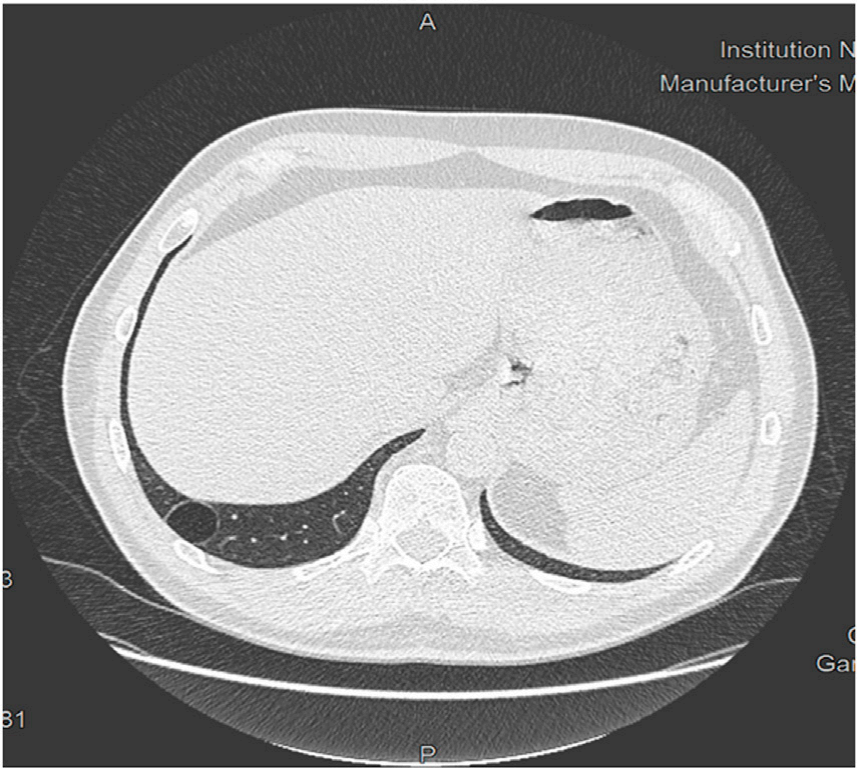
Chest CT scan showing a right basal subpleural cyst.
